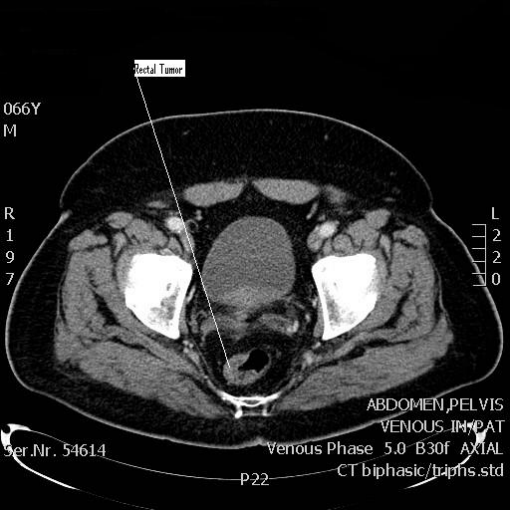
CT scan showing the thickenned wall of the rectum suggesting the presence of rectal carcinoma.
